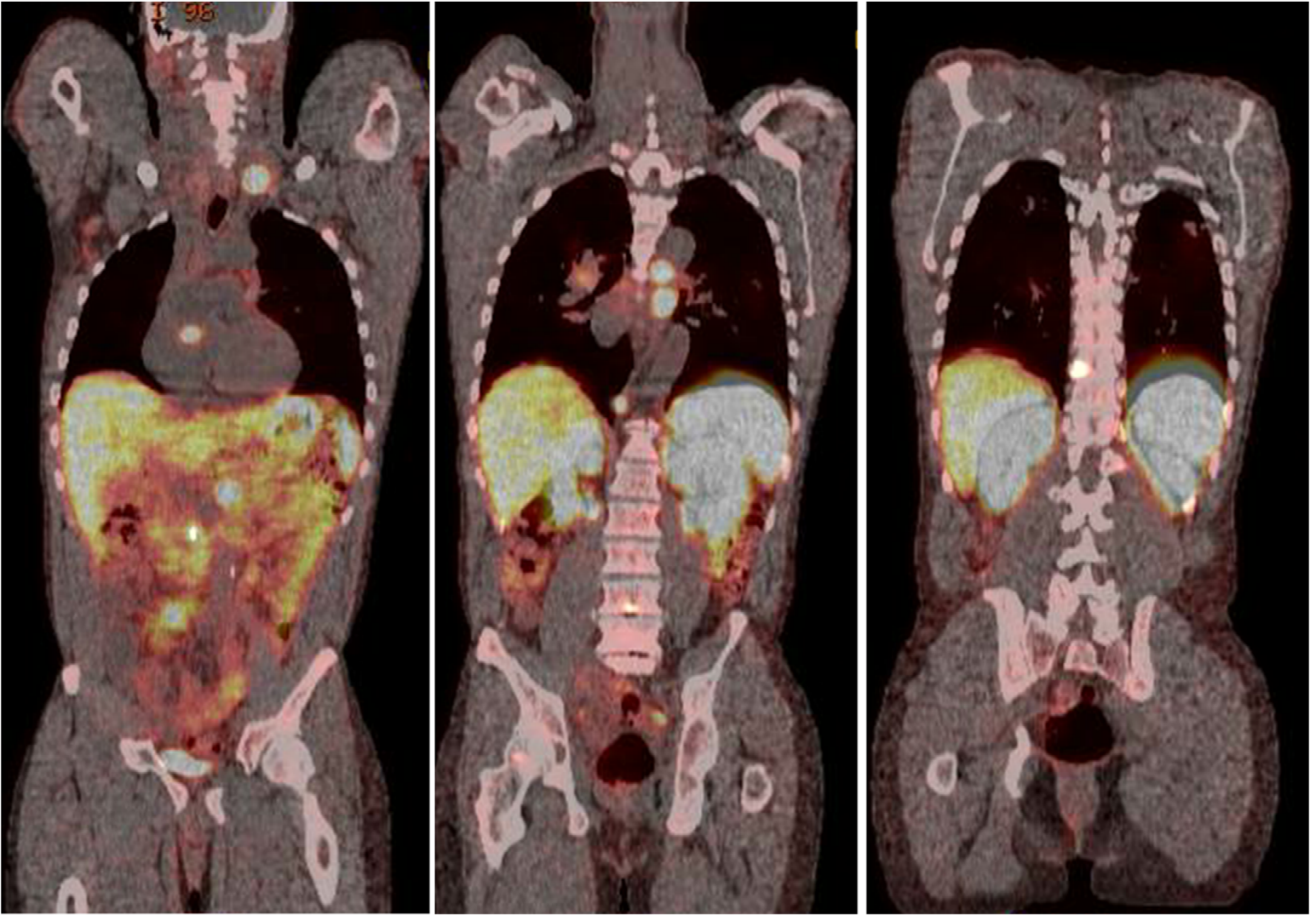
Gallium‐68 Dotatate positron emission tomography (PET)/computed tomography (CT) scan demonstrated metabolically active adenopathy involving the supraclavicular, mediastinum, upper abdomen, and retroperitoneal locations, as well as metabolically active left liver lobe, right small bowel mass, central calcified mesenteric mass, left and right lung nodules, and several lytic metastases in bone.
